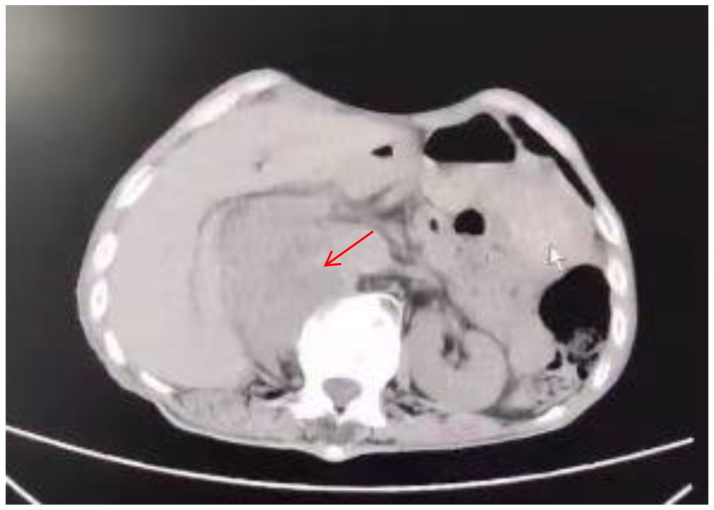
Follow-up abdominal CT shows adrenal metastasis. CT = computed tomography.
